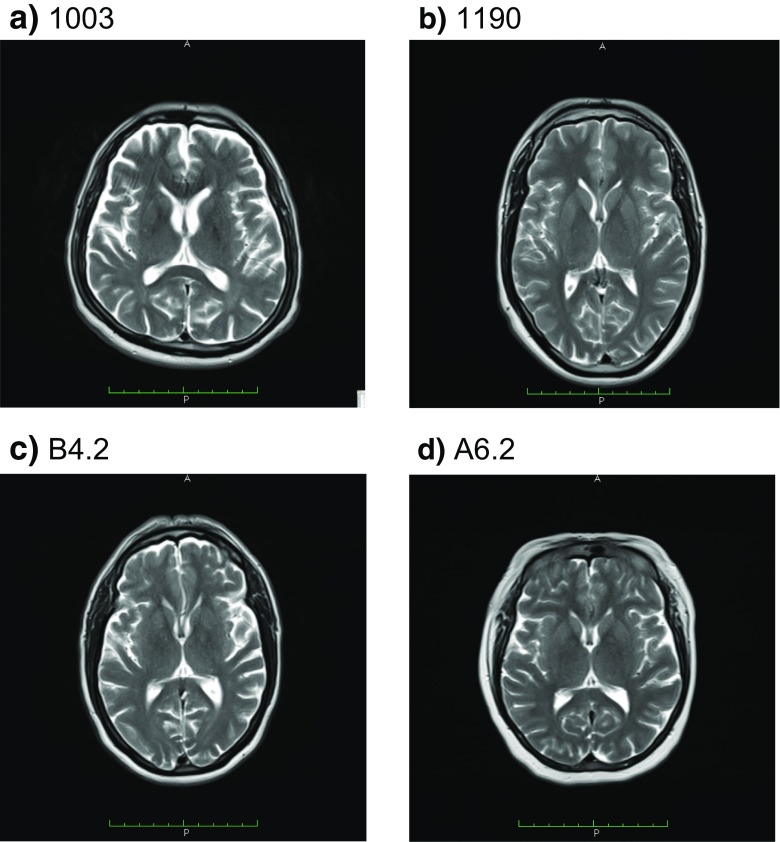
Axial T2-weighted brain MRI of the four individuals at a ganglionar level shows no brain structures lesions. a CMT1003. b CMT1190. c B4.2. d A6.2
